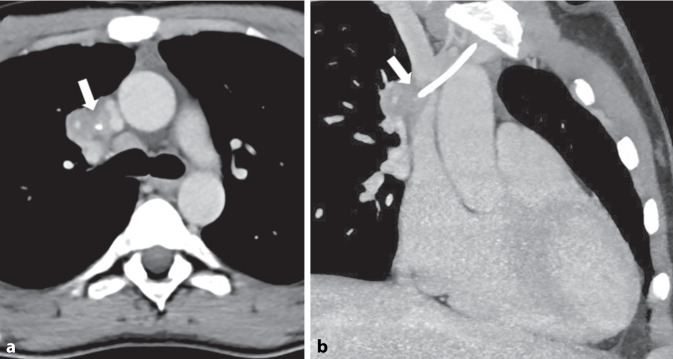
Computertomographie (*CT*) des Thorax mit axialer (a) und parakoronarer (b) Rekonstruktion im Weichteilfenster. Um die Spitze des Portkatheterschlauchs ist ein Thrombus abgrenzbar (*Pfeile*) mit angrenzendem Hämatom. Die Spitze des Portkatheters scheint neben der Vena cava superior zu liegen
